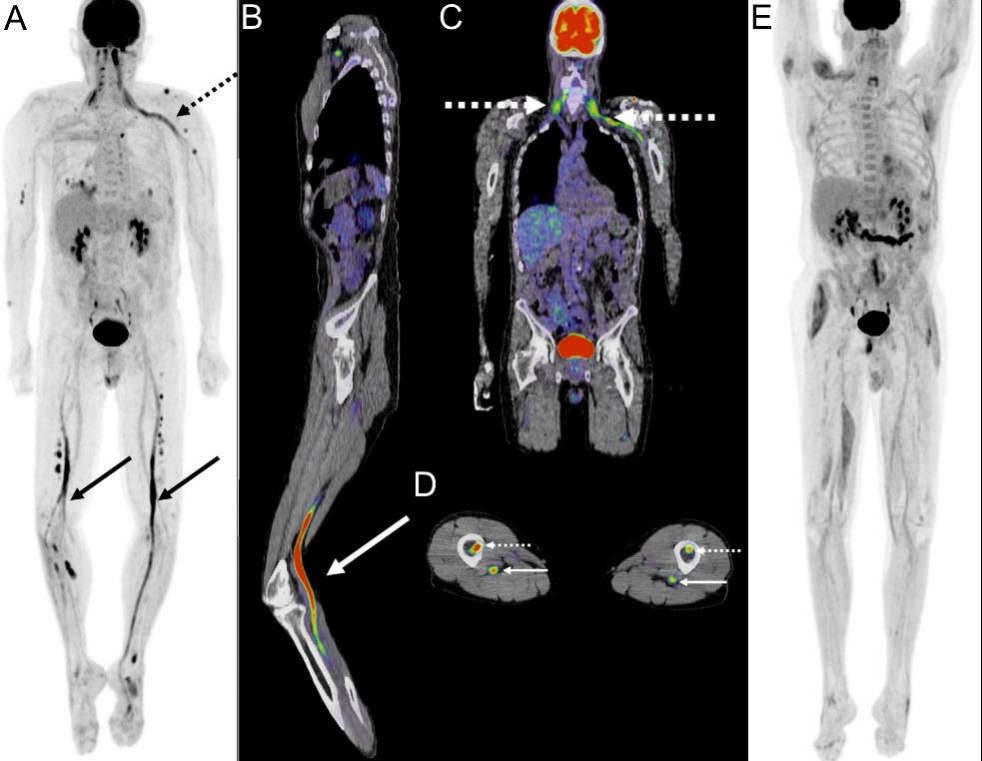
^18^F-FDG PET/CT whole-body maximum intensity projection image (A) shows linear abnormal uptake along the left brachial plexus (broken arrow), and in the bilateral sciatic nerves (arrows). Fused ^18^F-FDG PET/CT images demonstrate extension into the left tibial nerve (B arrow), involvement of the left cervicothoracic nerve roots and brachial plexus (C, dashed arrows), and osseus infiltration of the tibiae (D, broken arrows) and nearby neurovascular bundles (D, arrows). Follow-up ^18^F-FDG PET/CT performed 4 months later demonstrated near complete resolution at these sites (E). Asymmetric muscle uptake may be due to asymmetric muscular strain
